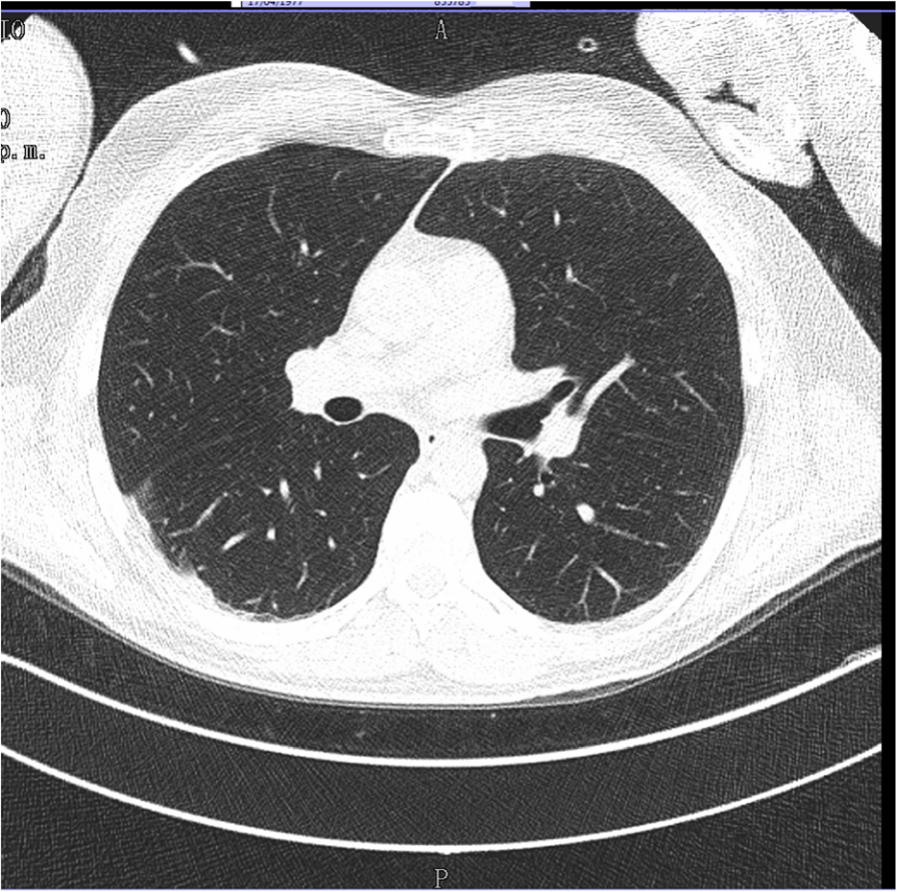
Chest CT scan not showing any infiltrates, consolidation images, or other signs of pneumonia; a nonsignificant single pleural nodule was identified
